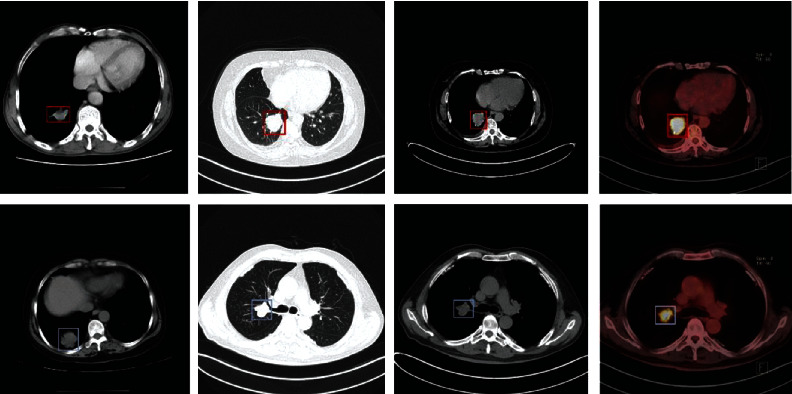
A large-scale lung cancer detection dataset for CT and PET/CT from the TCIA dataset.
